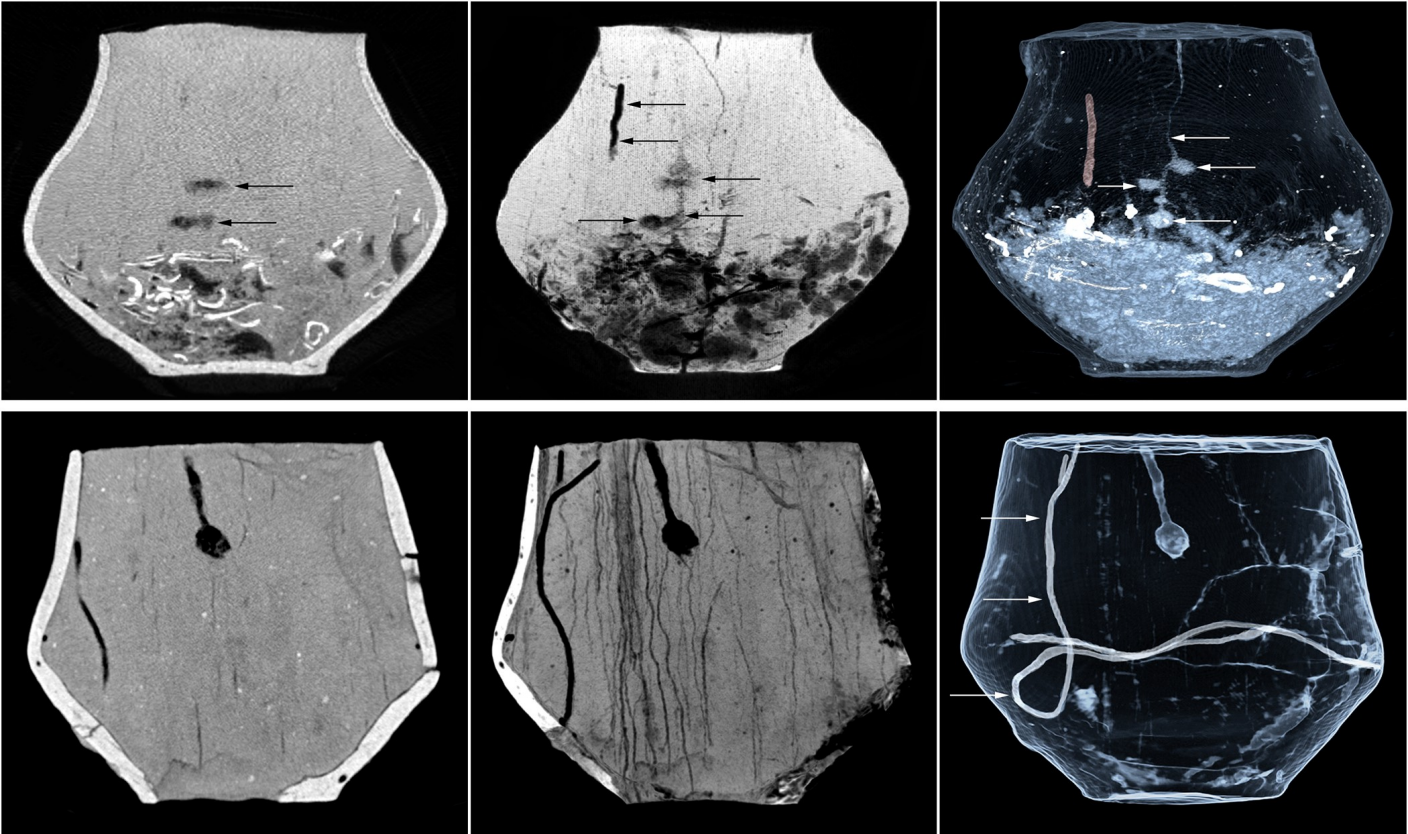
The CT imaging of the fill of the urn from grave 485 and the fill of the additional vessel from grave 600. At the top, the visualization of the urn fill from grave 485 with a preserved corridor of an insect of the genus *Harpalus* sp. and a disappearing nest of a beetle of the genus *Geotrupes* sp. At the bottom, the imaging of the interior of the additional vessel from grave 600 with an earthworm tunnel and an aestivation chamber of a different species of earthworm marked with arrows.
